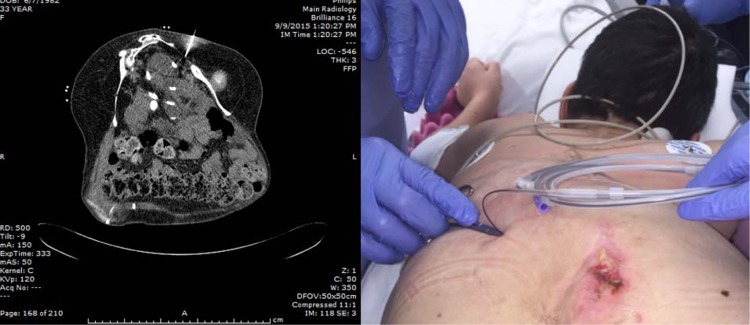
From *left* to *right*: Needle placement through left sciatic foramen using CT guidance; hydrophilic wire entering skin transgluteally to access left renal moiety (note sacral decubitus ulcer).
